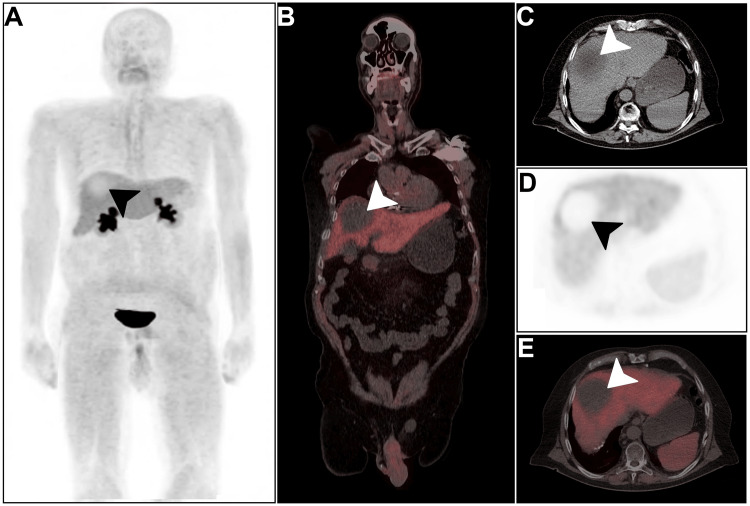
Baseline [68Ga]Ga-FAPI PET/CT (A-E) Maximum intensity projection [^68^Ga]Ga-FAPI, fused coronal, and axial PET/CT views depicted the hepatocellular carcinoma lesion as photopenic segment VII hypodensity with otherwise unmarkable scan elsewhere (arrowheads). FAPI: Fibroblast activation protein inhibitor
